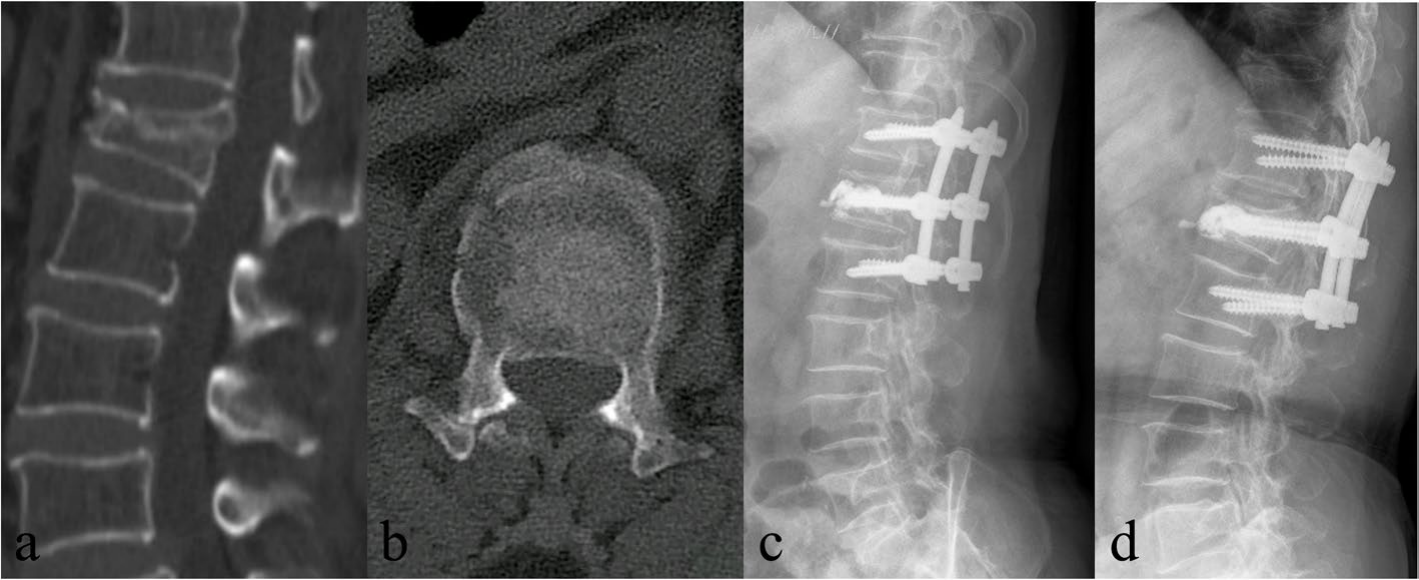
a-b Preoperative Sagittal and axial CT images of a 71-year-old female showing L1 burst fracture; c Immediate postoperative lateral plain radiograph showing AVH and kyphotic deformity were corrected after SSKF treatment; d Plain radiograph at the final follow-up
